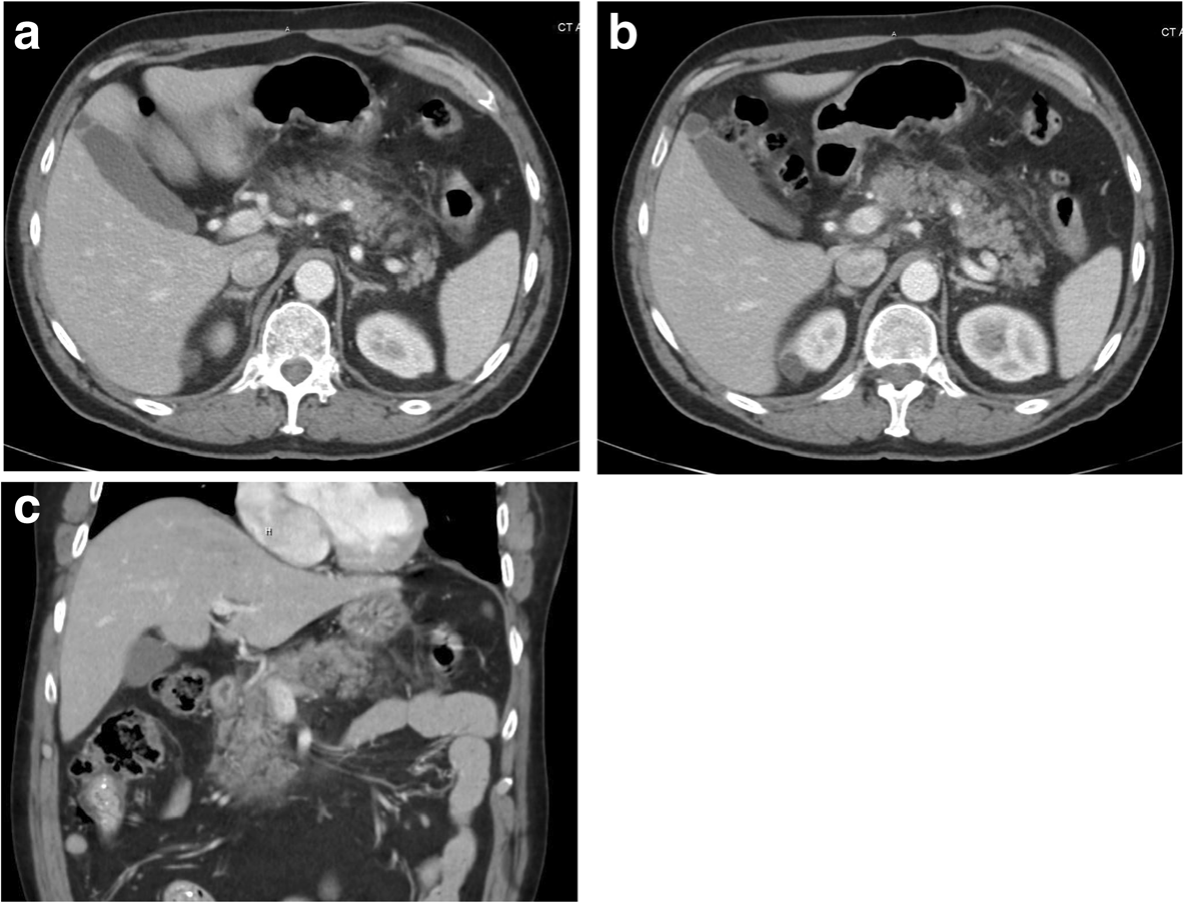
CT of the abdomen and pelvis. a and b Axial plane showing infiltration of the peripancreatic fat planes by soft tissue attenuation complicated with inflammation. No pancreatic ductal dilatation or discrete peripancreatic fluid collections observed. No stones in adjacent gall bladder. c Similar findings in coronal plane
